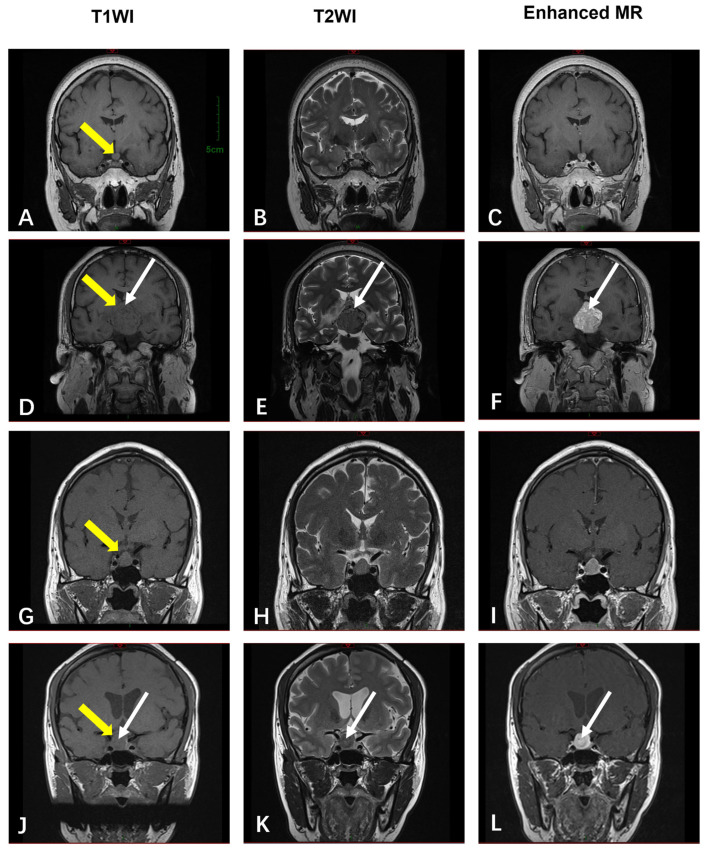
MRI images of 4 patients with sellar GCTs. (A,D,G,J) show that the 4 cases were isointense in T1WI (yellow arrows indicating the location of the lesions); (B,E,H,K) show variable signals in T2WI, ranging from isointense signals to low signals or slight hyperintensity; (C,F,I,L) show enhancement in all 4 cases. Note that within the masses, there are star-like cracks (white arrows in D–F,J–L) that show hypointensity on T1WI and hyperintensity on T2WI. The star-like crack is enhanced after contrast. Other subgraphs in Figure 5 and subgraph A share one scale bar.
